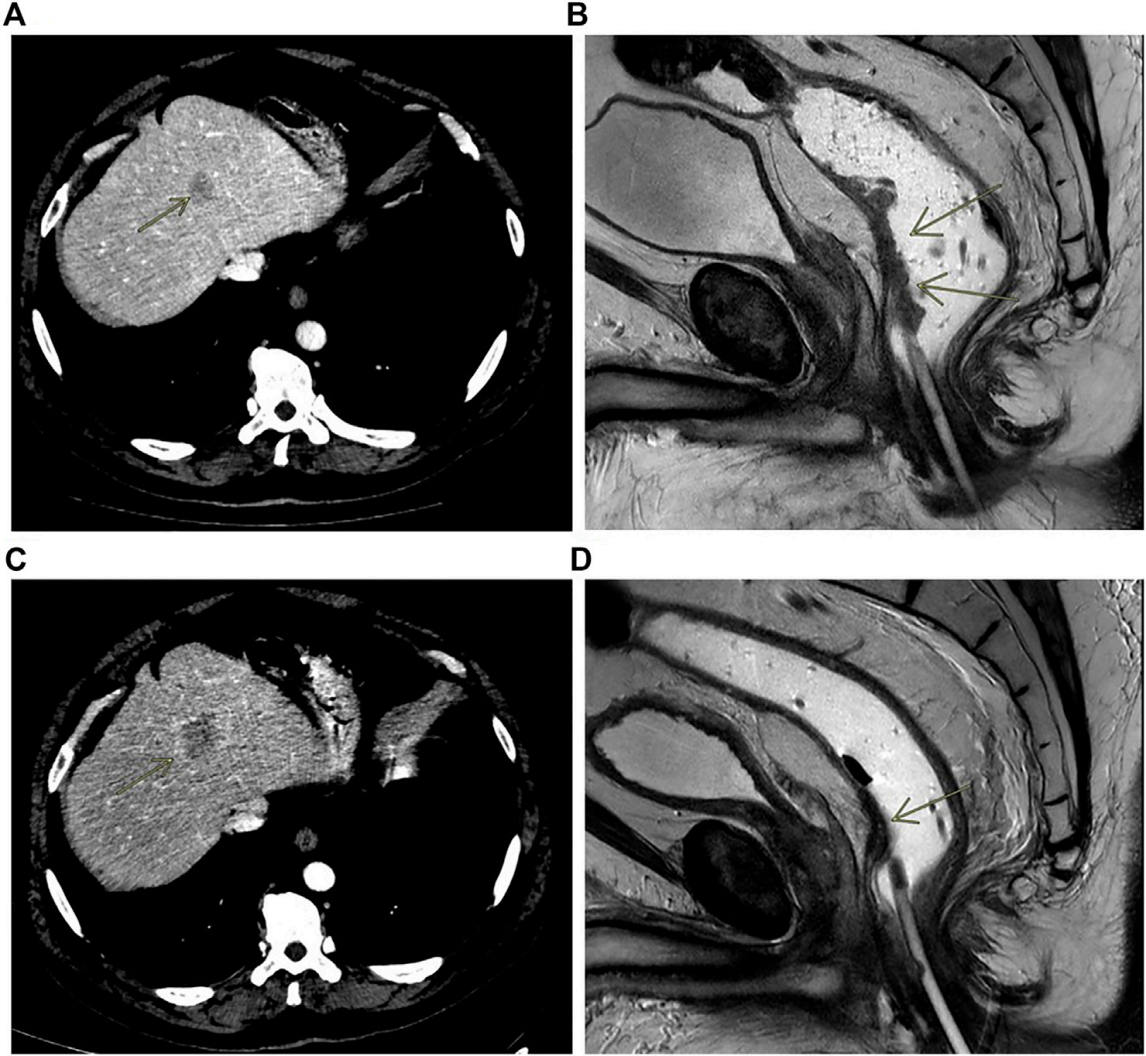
Contrast enhanced CT scan showing liver metastasis at baseline (A) and at progression (C); MRI rectal cancer at baseline (B) and after treatment (D).
